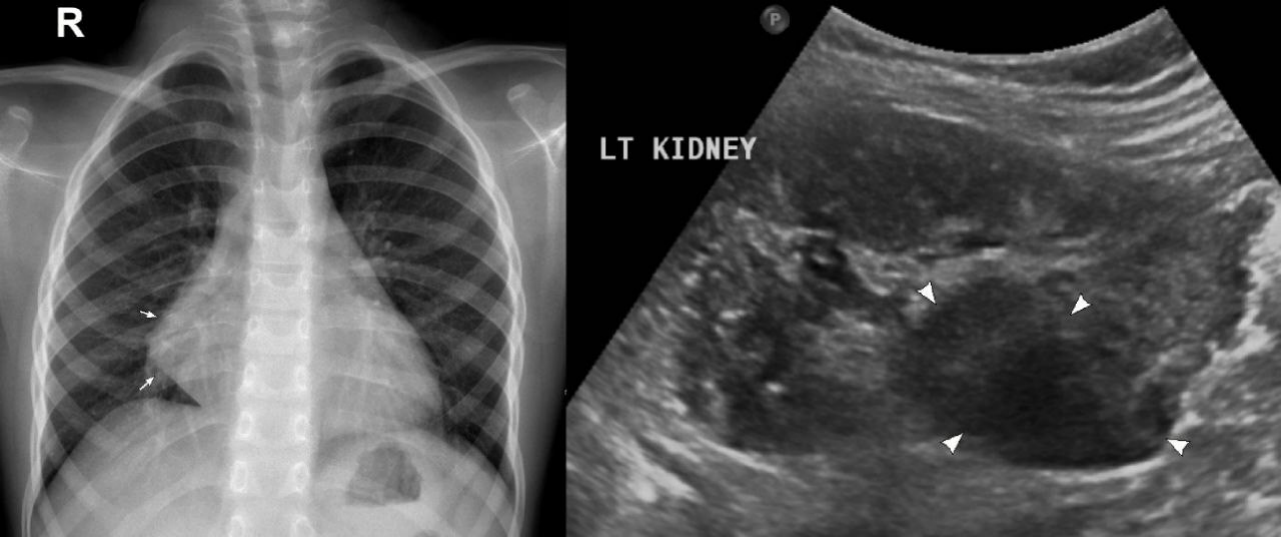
Follow-up chest X-ray and ultrasound of left kidney after approximately 2 years of medical treatment showing significant size reduction of the mediastinal (arrows) and renal (arrowheads) masses.
